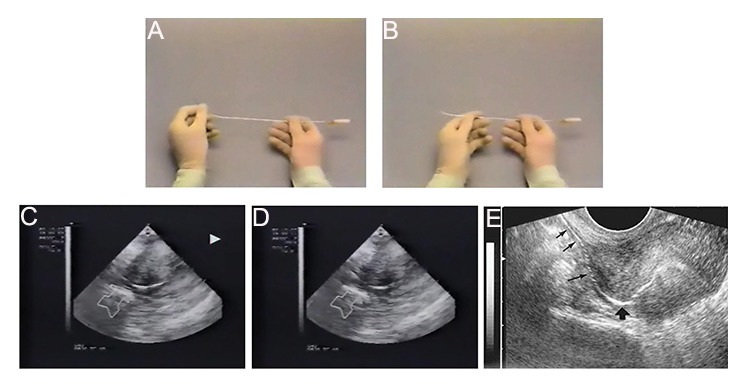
The cannula used and the ultrasound images of the ultrasound-guided tubal recanalization of proximal tubal obstruction (PTO): A.
